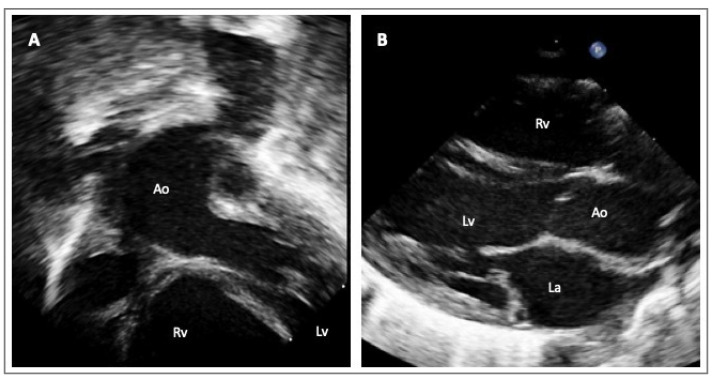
Echocardiographic images of a one-year-old boy with suspected LDS/MS presenting with an isolated dilatation of the ascending aorta. Subcostal view of the aorta (Panel A); parasternal long axis view (Panel B). Legend: left ventricle (Lv); right ventricle (Rv); aorta (Ao); left atrium (La).
